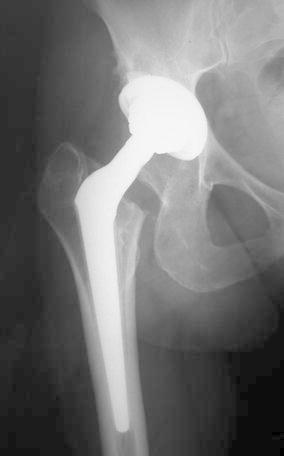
Radiographic aspect in anteroposterior view at 11 years follow-up: the implant is stable and no signs of bone resorption are noticeable
